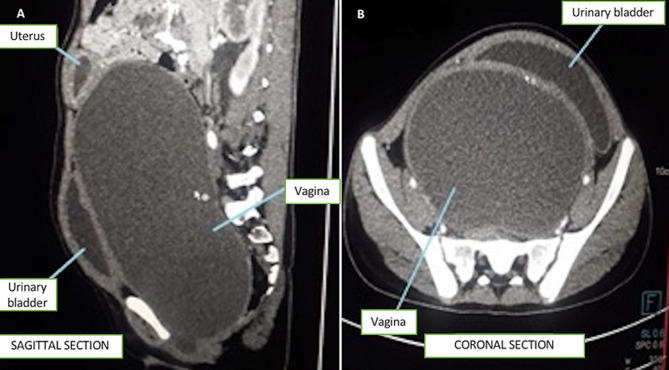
sagittal (A) and coronal (B) computed tomography shows a significantly distended vagina resulting in the compression of the urinary bladder
